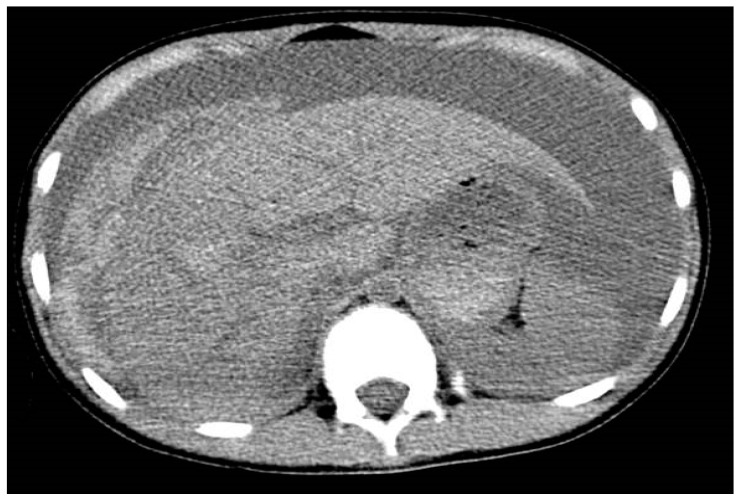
CT shows hematomas within the liver parenchyma, with rupture of the capsule and development of hemoperitoneum.
